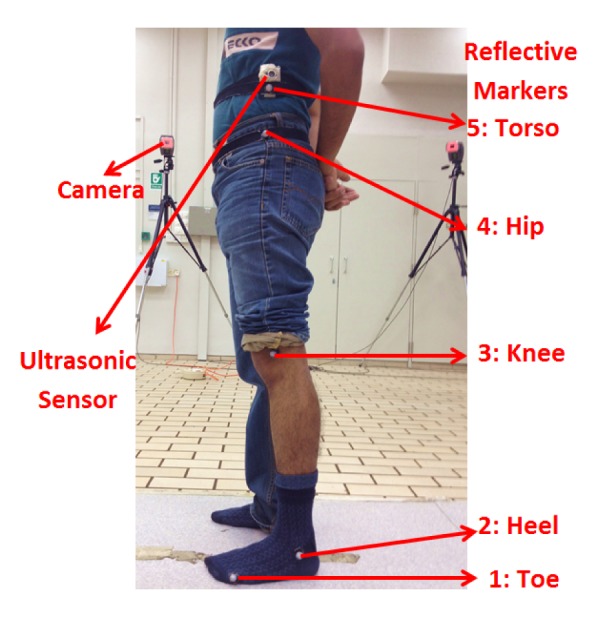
Wireless unit with embedded ultrasonic sensor placed on the lateral side of the trunk and five reflective markers fixed on the toe, heel, knee, hip and torso for the optical tracking reference system.
